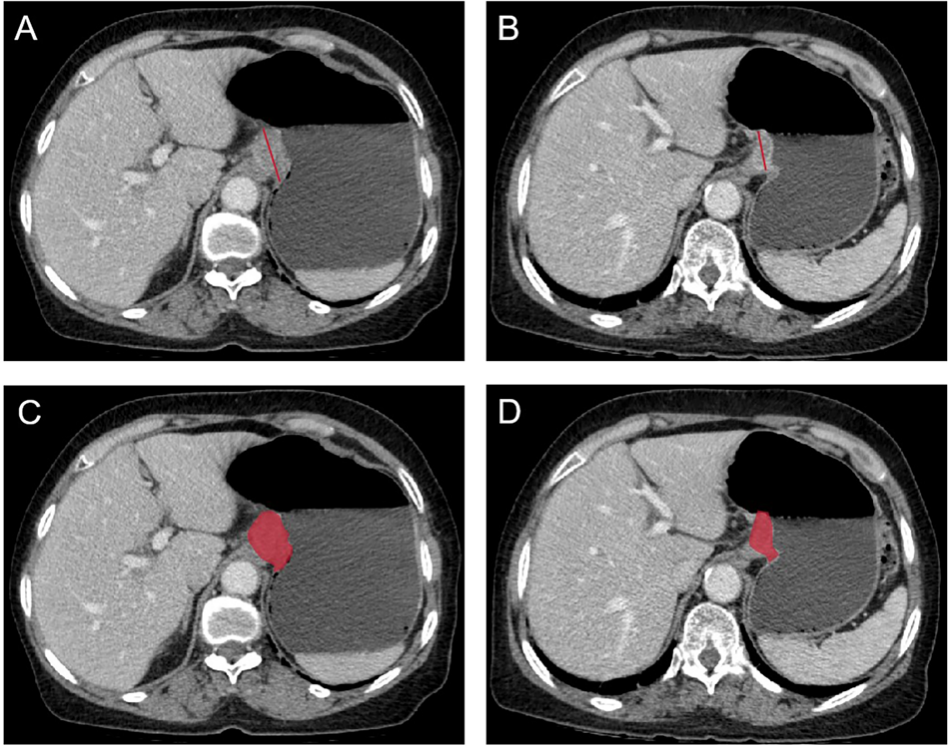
Advanced adenocarcinoma of esophagogastric junction in a 71-year-old man. A, B Show measurement of the tumoral longest diameter on portal venous phase axial CT image before and after neoadjuvant chemotherapy, respectively. C, D Depict segmentation of volume of interest on portal venous phase axial CT before and after neoadjuvant chemotherapy, respectively
